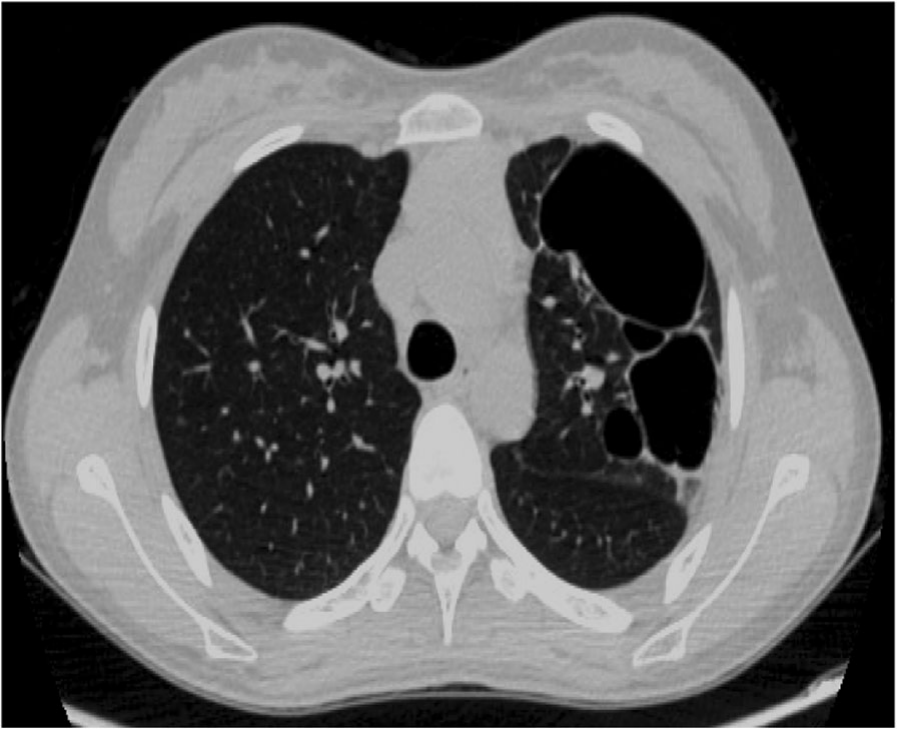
Computed tomography image 6 months after operation: cystic lesions did not improve
